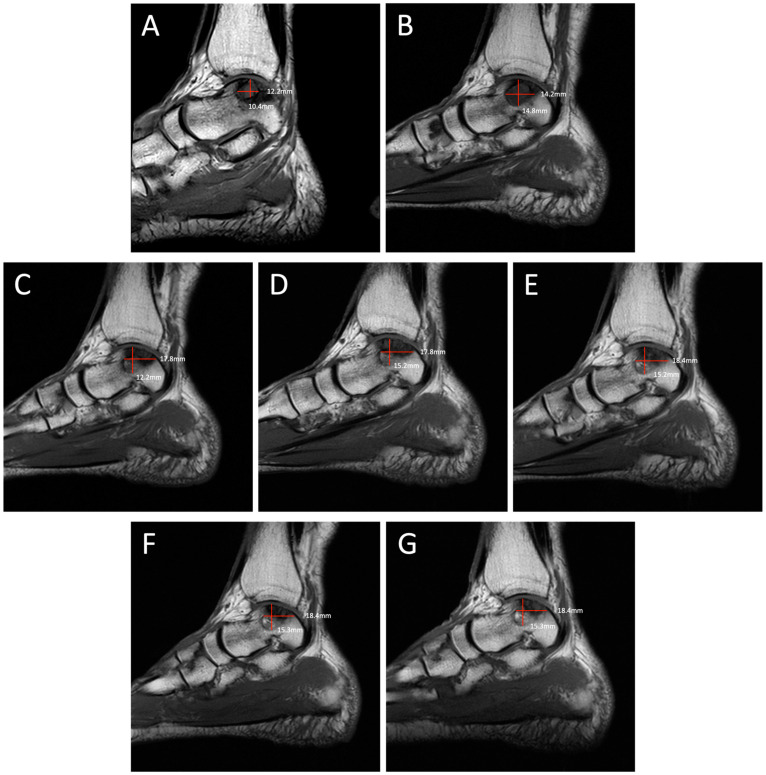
Degenerative progression of the left medial osteochondral lesion outlined in red on sagittal MRIs from 2009 to 2021. The timeline of lesion progression includes MRI scans from 2009 (A), 2011 (B), 2016 (C), 2017 (D), 2018 (E), 2019 (F), and 2021 (G). This sagittal view demonstrates the osteoarthritic progression of the lesion from 12.2 mm × 10.4 mm in 2009 to 18.4 mm × 15.3 mm in 2021.
